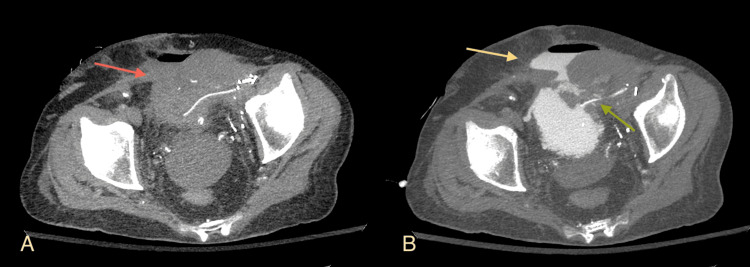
Double urine leak in a patient with a history of previous leak after kidney transplantation and Boari flap surgery (A) Multidetector computed tomography (MDCT) Maximum Intensity Projection (MIP) axial projection before retrograde administration of contrast medium indicates a hypodense fluid collection containing air adjacent to the bladder, involving the anterior lower abdominal wall; (B) MDCT MIP axial projection after the retrograde administration of contrast medium. There is a hyperdense small leak around the ureteric stent (green arrow) and a larger collection containing fluid and air in the lower anterior abdominal wall due to leak from the upper wall of the bladder (yellow arrow). Image source: Department of Radiology, Ippokrateio General Hospital of Thessaloniki. Permission obtained for reproduction.
